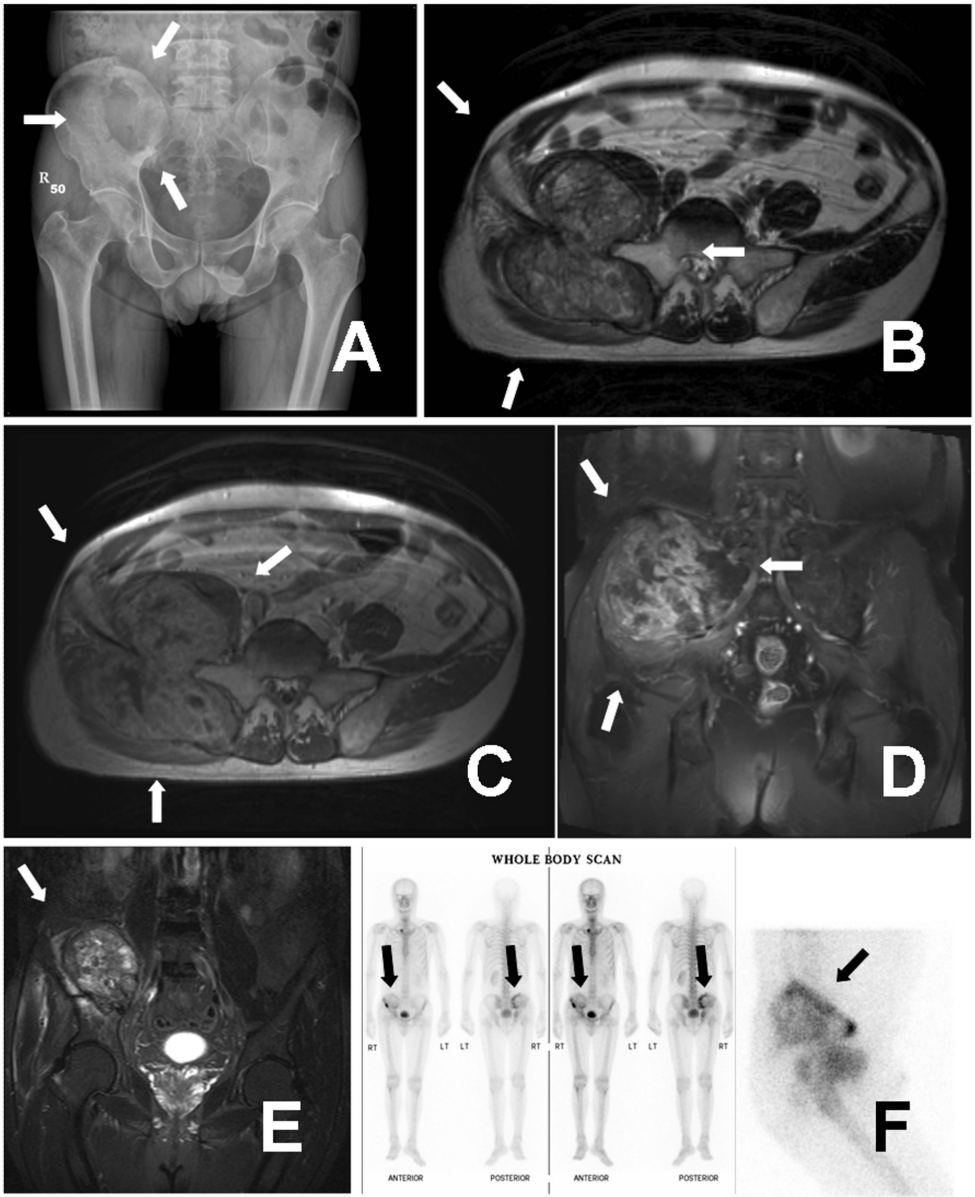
Pelvis radiograph showed a large osteolytic lesion over right iliac bone near sacroiliac junction (white arrows) (A). MRI revealed a 9.6 × 9.4 × 9.0 cm bilobular huge tumor mass arising from right iliac wing with bony destruction and significant soft tissue extension (white arrows) (B to E). ^99m^Tc-MDP BS showed mildly hot areas at right iliac bone with a large, round cold area (black arrows) (F). B: axial T1-weighted image with contrast enhancement. C: axial T2-weighted image. D: coronal fat-suppressed (FS) T2-weighted image with contrast enhancement. E: coronal short tau inversion recovery (STIR) images.
